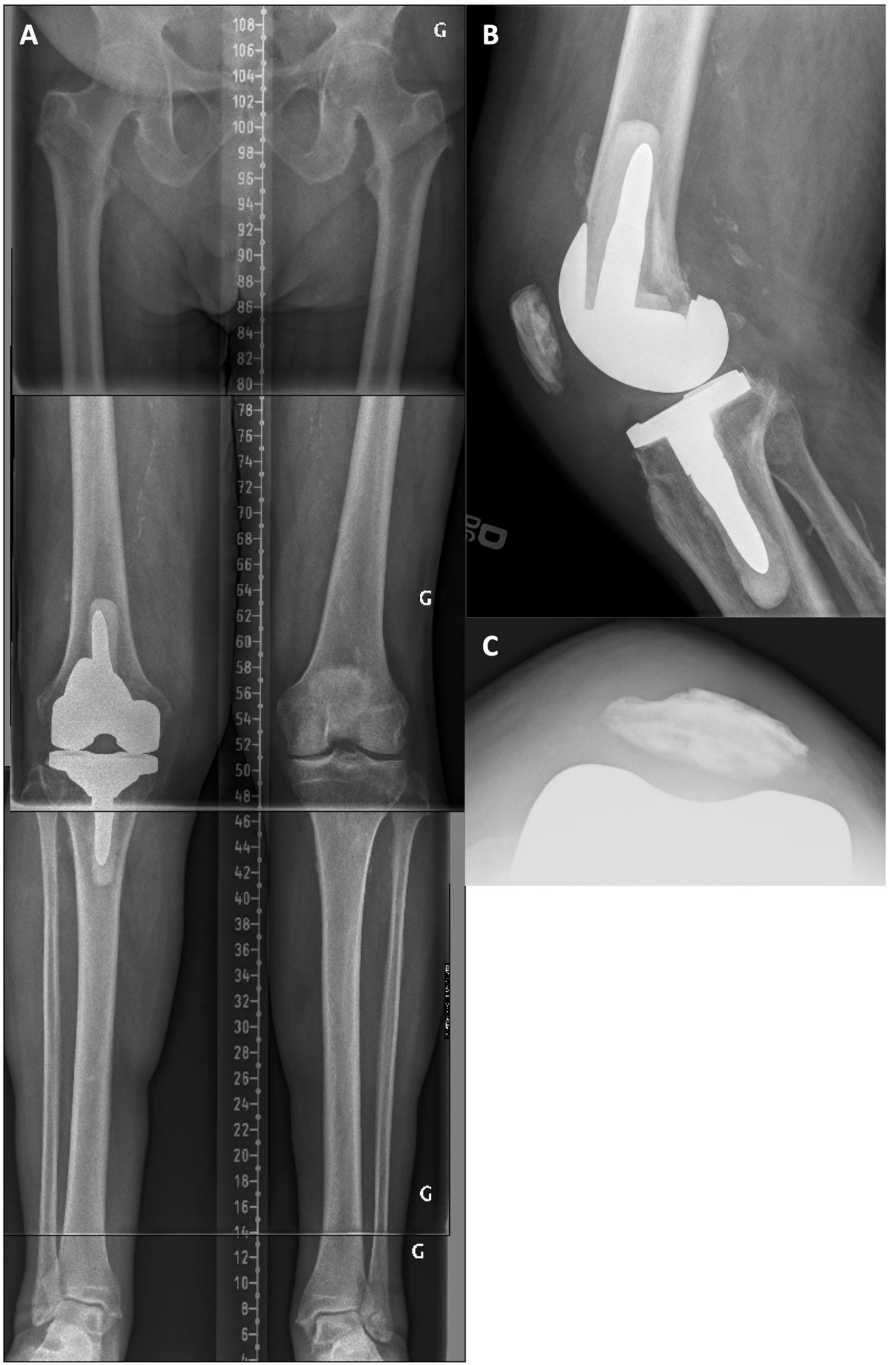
(A) Post-revision standing long-leg X-ray of the right lower limb where the implant mDFA has been modified to 1.0° valgus and the mPTA at 0.5° varus (0.5° valgus aHKA). (B) Right knee lateral view where the implant tibial slope has been shifted from 1.5° anterior to 2.0° posterior. The femoral implant has also been translated posteriorly to be flush with the anterior cortex. (C) Right knee skyline view showing a well-centered resurfaced patella.
